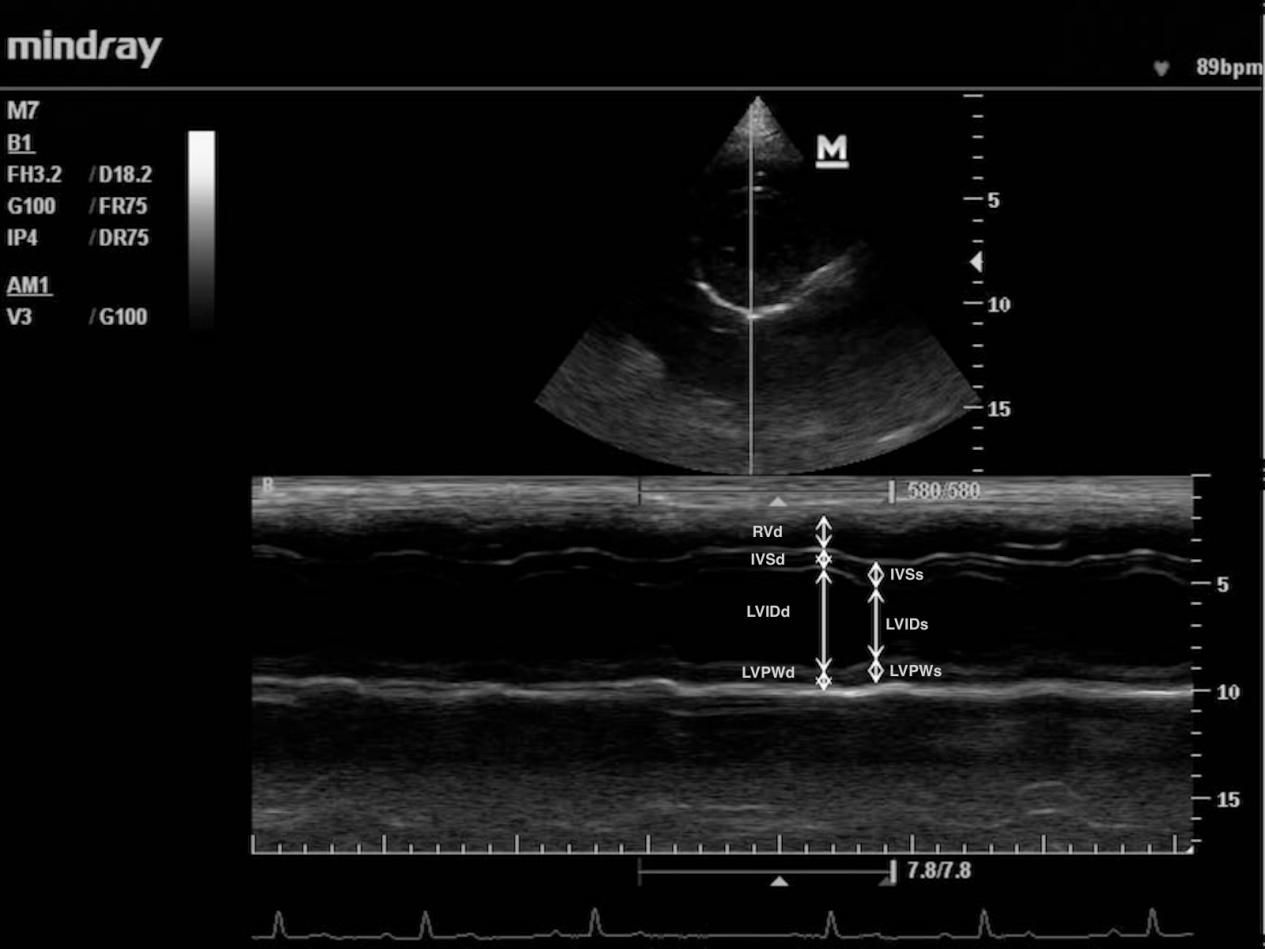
M-mode of the right and left ventricle obtained from the right parasternal short-axis view at the level of papillary muscles. RVd- right ventricle, IVSd- inter-ventricular septum, LVIDd—left ventricular internal diameter, LVPWd- left ventricular posterior wall thickness in diastole respectively, IVSs- inter-ventricular septum, LVIDs—left ventricular internal diameter, LVPWs- left ventricular posterior wall thickness in systole respectively.
